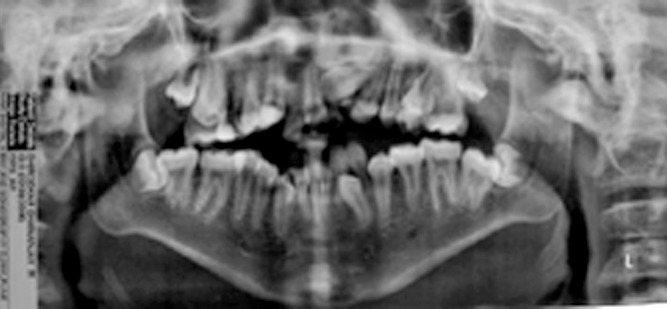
Panoramic radiograph showing over retained teeth
